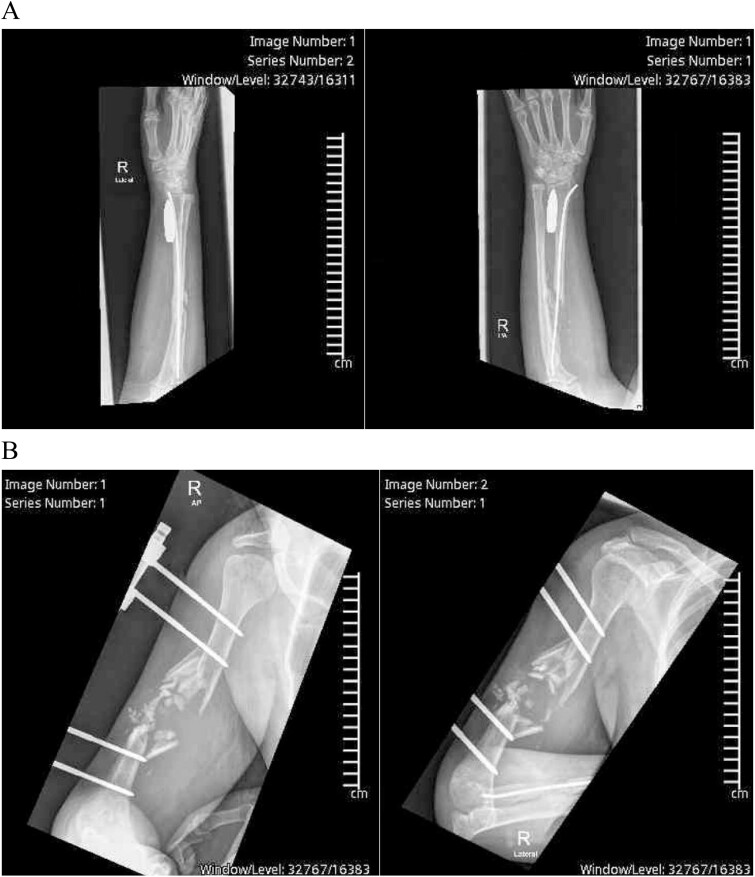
(A) Preoperative X-ray showing the bullet lodgment along the volar aspect of the distal radius. (B) Preoperative X-ray showing the external fixation device with pins at the proximal and distal ends of the right humerus with shattered mid-third of the shaft, surrounded by displaced fracture fragments, which was shattered along the bullet’s tract before settling in the wrist.
